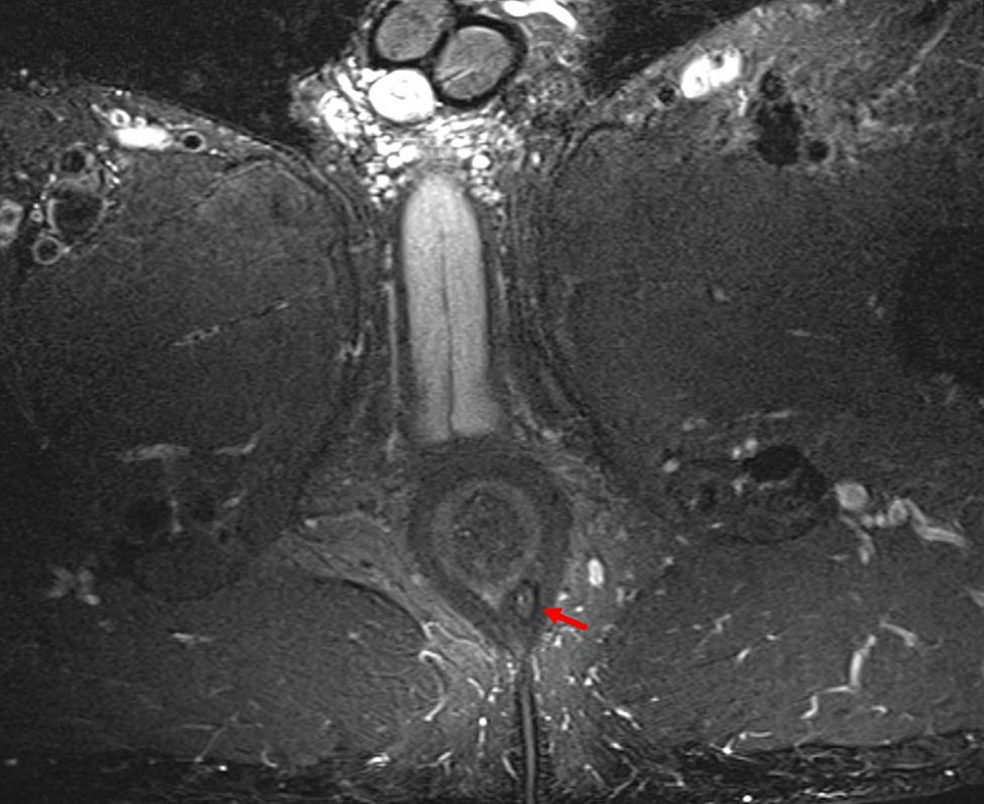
MRI of perianal fistula Axial, T2-weighted MRI image showing fistulous tract adjacent to the left posterior aspect of the rectum (arrow).
